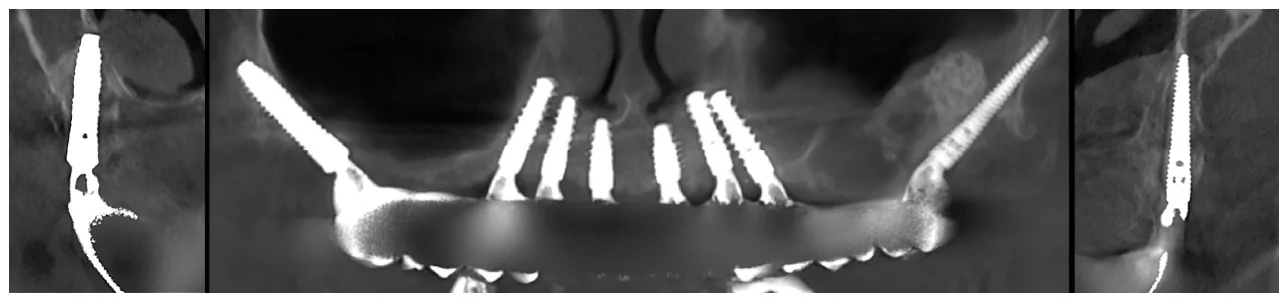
Follow-up CBCT, showing both definitive pterygoid implants correctly positioned and osseointegrated (right: Straumann TLC 4.5 × 18 mm; left: Southern Implants 4.0 × 24 mm, placed trans-sinus), with the anterior implants in function.
